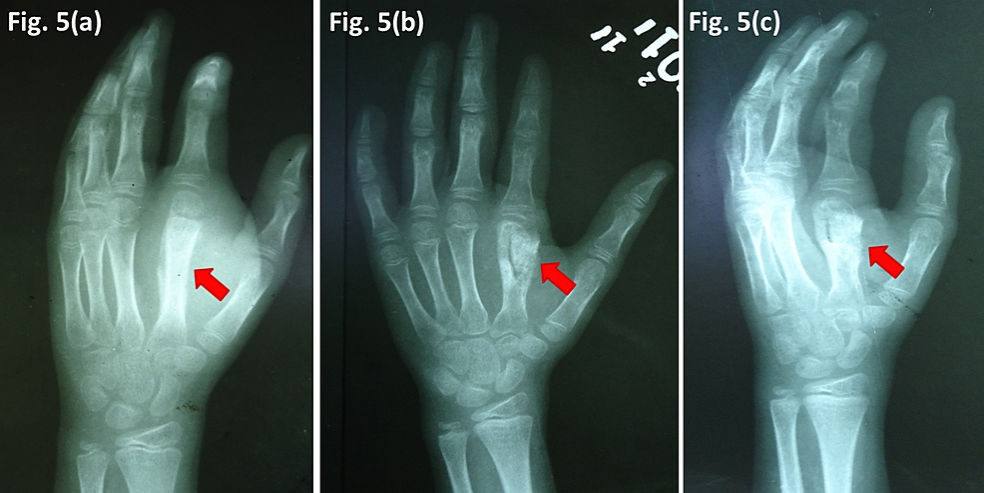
TB of metacarpal and phalanx (a) Plain radiograph taken elsewhere three months before presentation (b) At two months post ATT (c) After 12 months ATT TB - tuberculosis, ATT - anti-tubercular drug therapy
